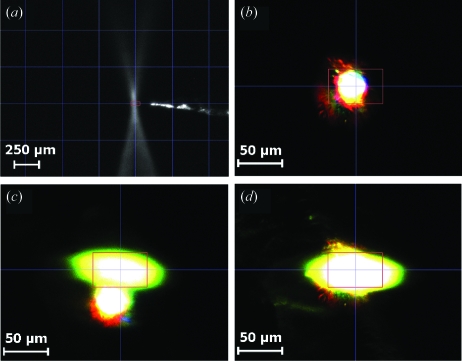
(*a*) Alignment of the focal point of the xenon lamp to the focal plane of the alignment camera using a camera mounted directly above the beamline and a pin at the sample position; the focus of the Schwarzschild objective can be clearly seen. The placement of a scintillator at the sample position allows the sample alignment camera to be used for alignment of the X-ray and optical axes: (*b*) X-ray shutter closed, optical shutter open; (*c*) both X-ray and optical shutters open with objective mis-aligned; (*d*) both X-ray and optical shutter open with the position of the yaw of the objective mount adjusted to maximize overlap of the X-ray and optical beams at the sample position.
